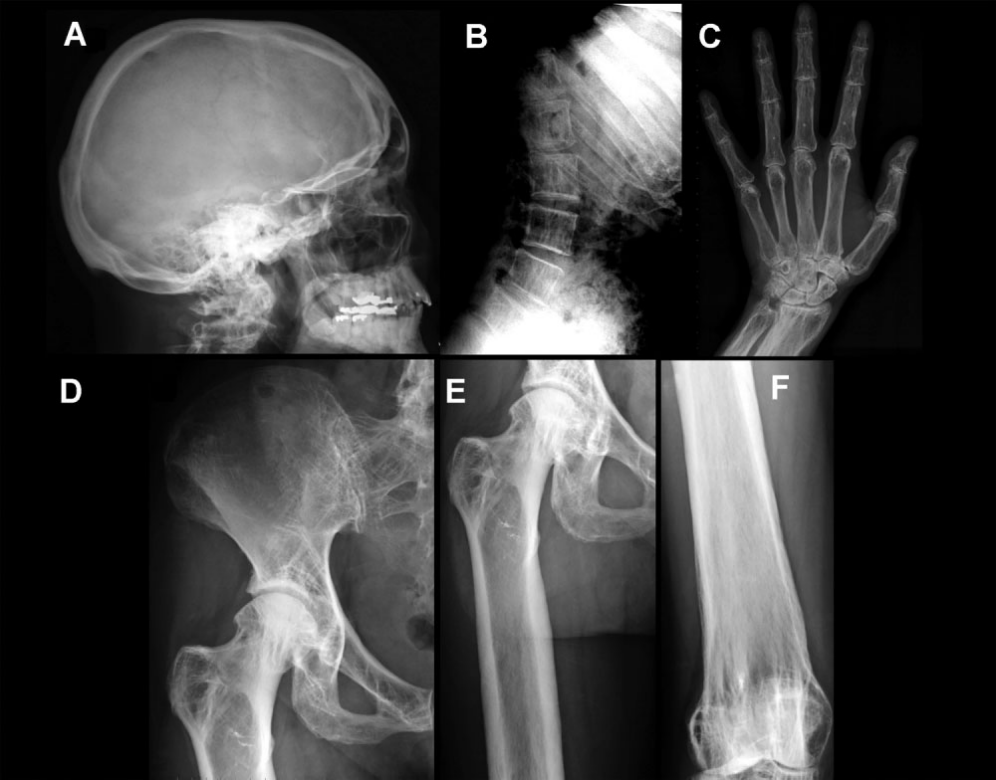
Radiographic findings of Patient 2 (mother) (*A-F*) The radiographic skeletal findings are in all ways similar to those of the son but are generally less severe. The skull is normal, with no osteosclerosis and a normal medullary space. The overall pattern of osteosclerosis and bone expansion is present, although there is less prominent vertebral body change and better tubulation of the long bones and less prominent trabecular thickening. There is less immature periostitis noted along the long tubular bones, although the mature periosteal reaction is again seen to extend to the epiphysis. As in the son, there are changes of moderate bilateral hip osteoarthritis and early lumbar degenerative disk disease.
